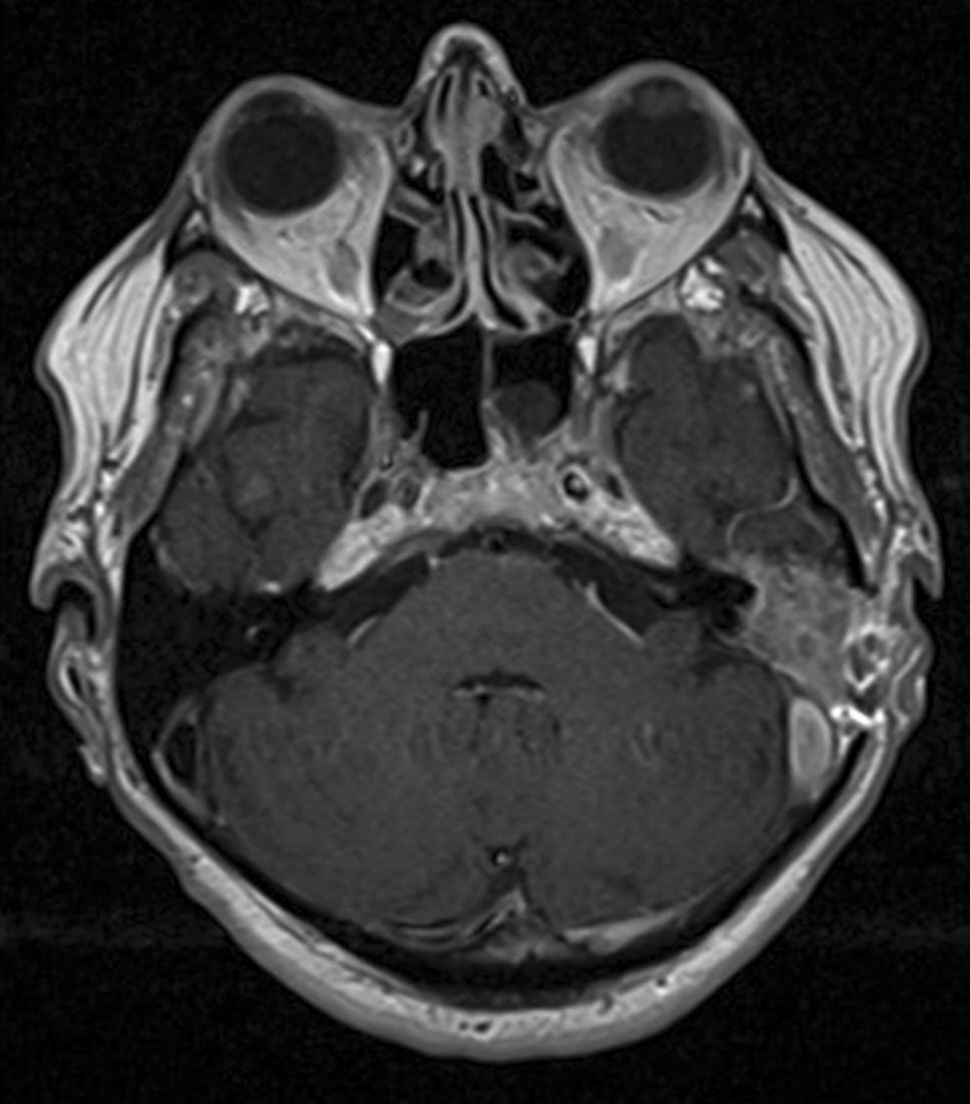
Axial T1-weighted MR images demonstrating an enhancing soft-tissue formation in the mastoid process and thrombosis of the sigmoid sinus
